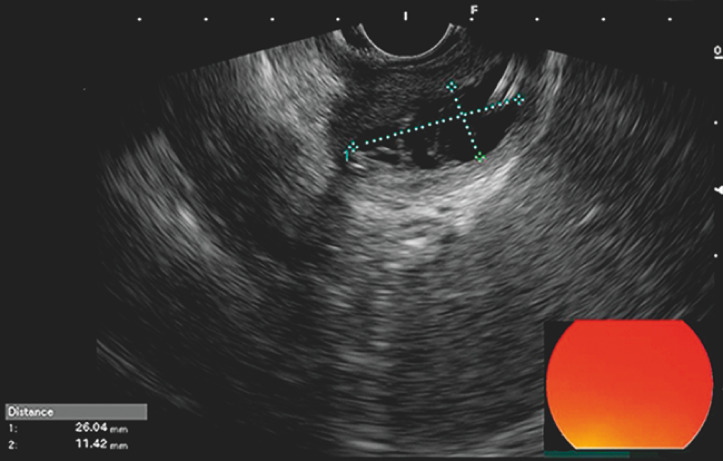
Endoscopic ultrasound after 2 weeks of drainage showed shrinking of the pseudocyst to approximately 26 × 11 mm.
